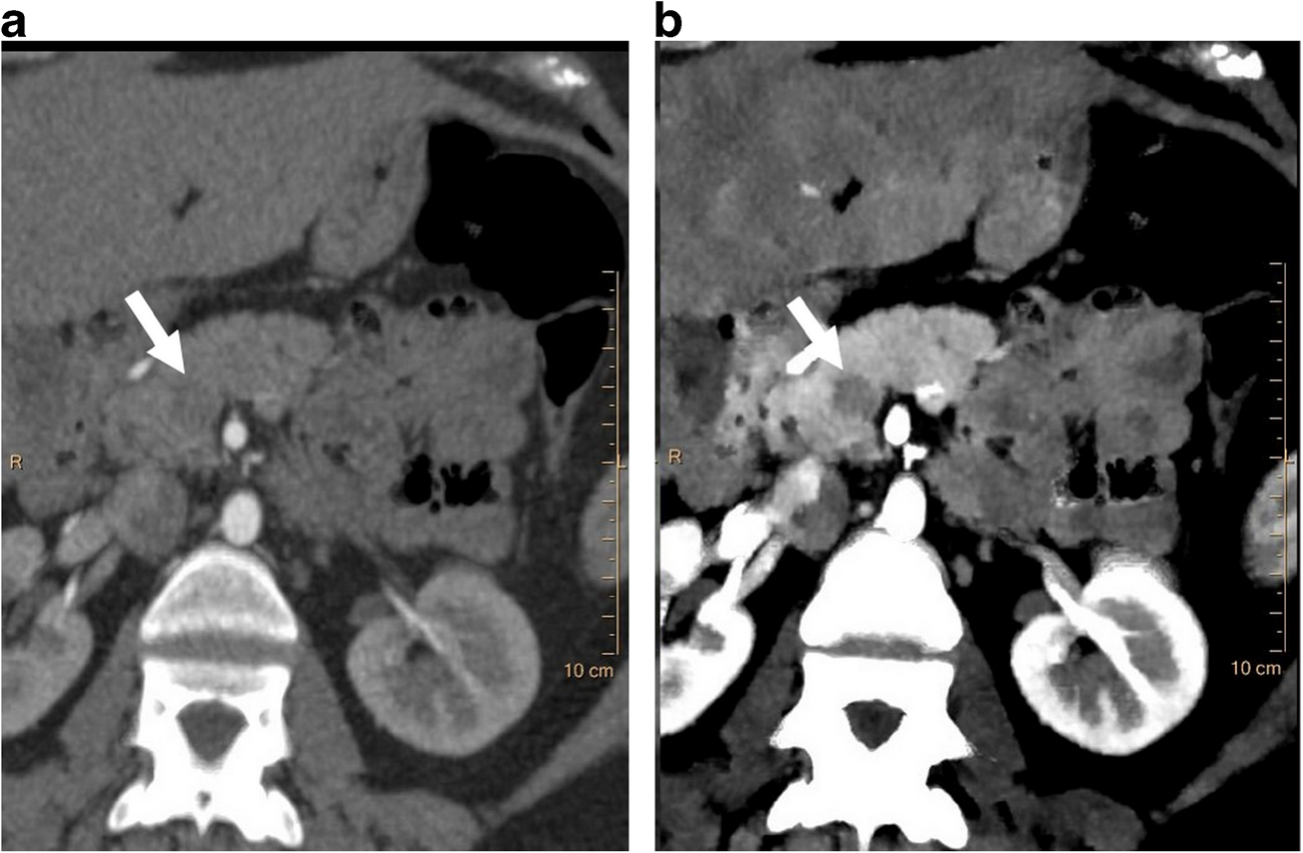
Improved lesion conspicuousness. (a) Axial 120-kVp routine diagnostic CT image through the upper abdomen shows a vague ill-defined hypoattenuating lesion in the pancreatic head (*arrow*). (b) 50-keV VMI at the same level shows excellent contrast opacification of the pancreas, with much improved conspicuity of the hypoattenuating pancreatic head lesion (*arrow*)
